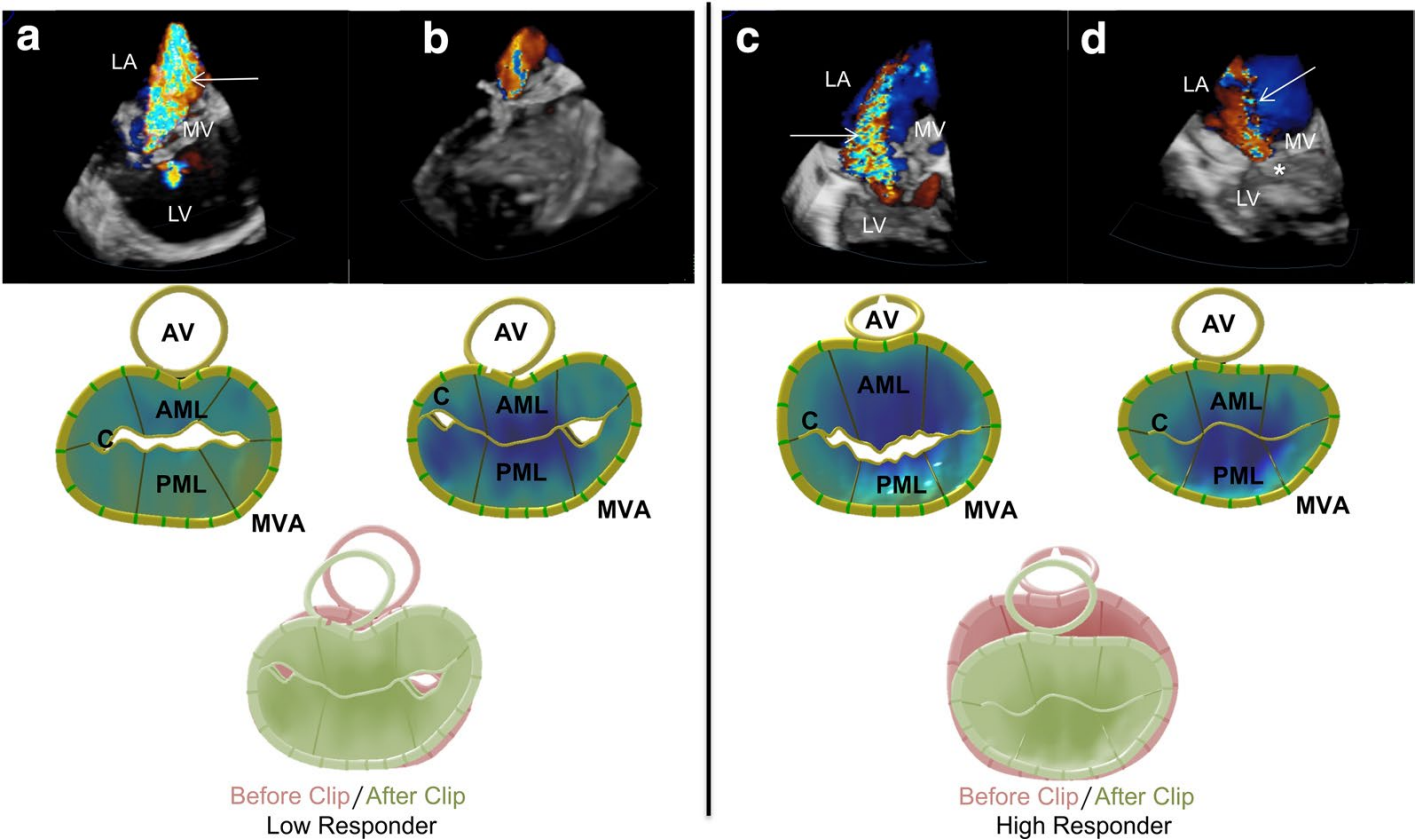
Exemplary 3D models of the acute effects of TMVR on the mitral valve of Low Responder (left) and High Responder (right). Upper picture: Overview of three-dimensional transesophageal echocardiography images comparing Low and High Responder before and after TMVR. Mitral regurgitation jet is visible (right arrow) as well as the MitraClip^®^ device (asterisk) itself. a 3D TEE image of Low Responder before TMVR showing severe MR. b 3D TEE image of Low Responder after TMVR showing mild MR. c 3D TEE image of High Responder before TMVR showing severe MR. d 3D TEE image of High Responder after TMVR showing mild MR. Lower picture: Left: 3D model of mitral valves (MV) before and after TMVR of Low Responders with corresponding colored models placed on top of each other to demonstrate change in annular size. Model of MV before and after clip with little change in annular size. Right: Model of Qlab MV before and after TMVR. Change in annular size is more prominent here. *AML* anterior mitral leaflet, *AV* aortic valve, *C* circumference, *LA* left atrium, *LV* left ventricle, *MV* mitral valve, *MVA* mitral valve annulus, *PML* posterior mitral leaflet, *target* indicates mitral regurgitation jet, asterisk indicates clip
